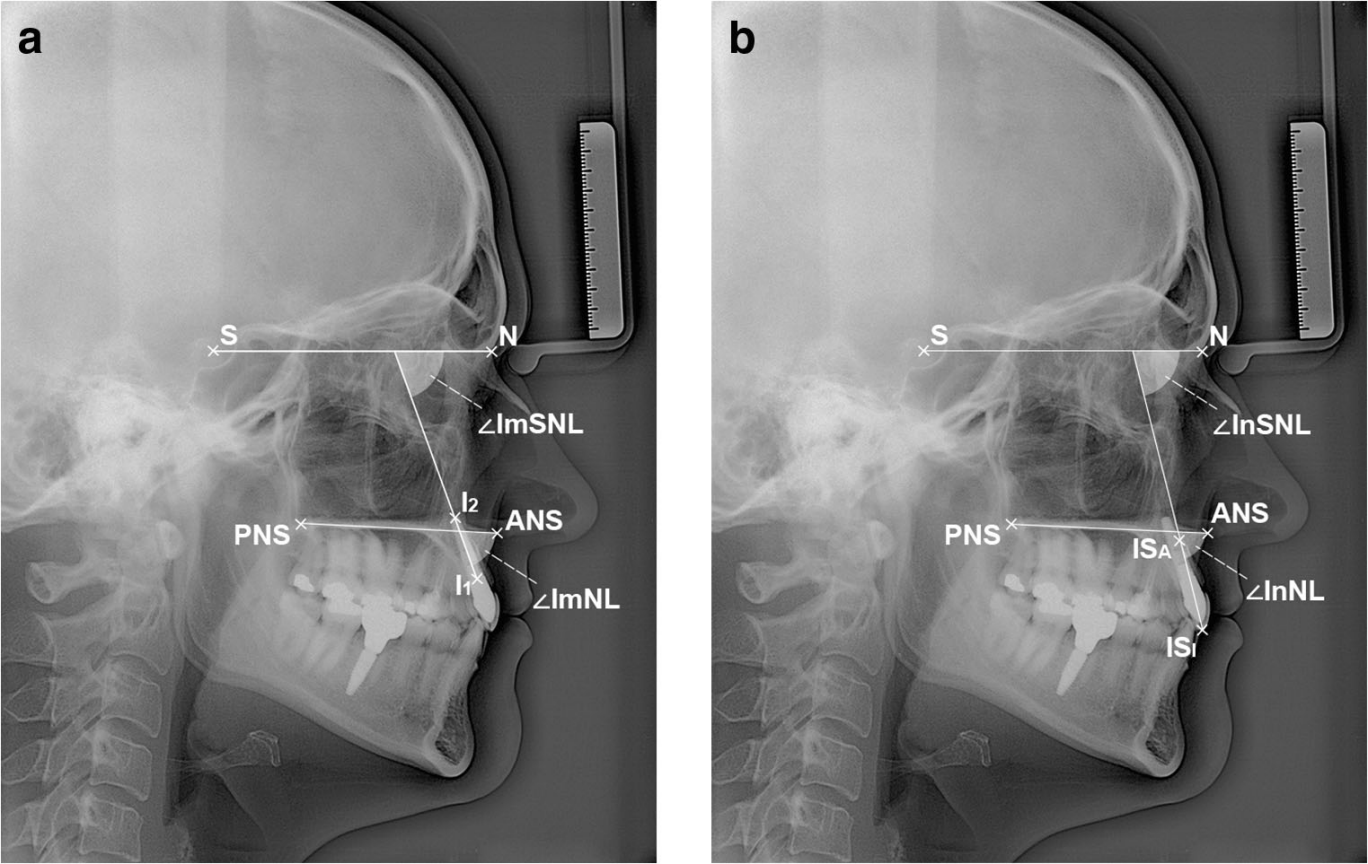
Radiographic parameters. A Implant angles. ANS anterior nasal spine, I1 implant shoulder, I2 implant apex, N Nasion, PNS posterior nasal spine, S Sella, ∠ImNL implant-NL angle, ∠ImSNL implant-SNL angle. B Incisor angles. ANS anterior nasal spine, ISA incision superius apicale, ISI incision superius incisale, N Nasion, PNS posterior nasal spine, S Sella, ∠InNL incisor-NL angle, ∠InSNL incisor-SNL angle
